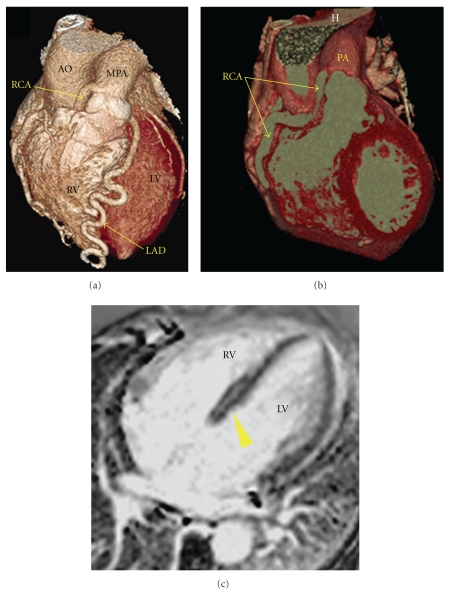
Patient no. 1: (a) Volume-rendered computed tomography shows the anomalous origin of the right coronary artery from the main pulmonary artery. (b) Full length of the dilated RCA. (c) Late postgadolinium CMR imaging demonstrates enhancement of the mid-interventricular septum (arrowhead) consistent with fibrosis. AO: aorta, LAD: left anterior descending coronary artery, LV: left ventricle, MPA: main pulmonary artery, RCA: right coronary artery, RV: right ventricle.
